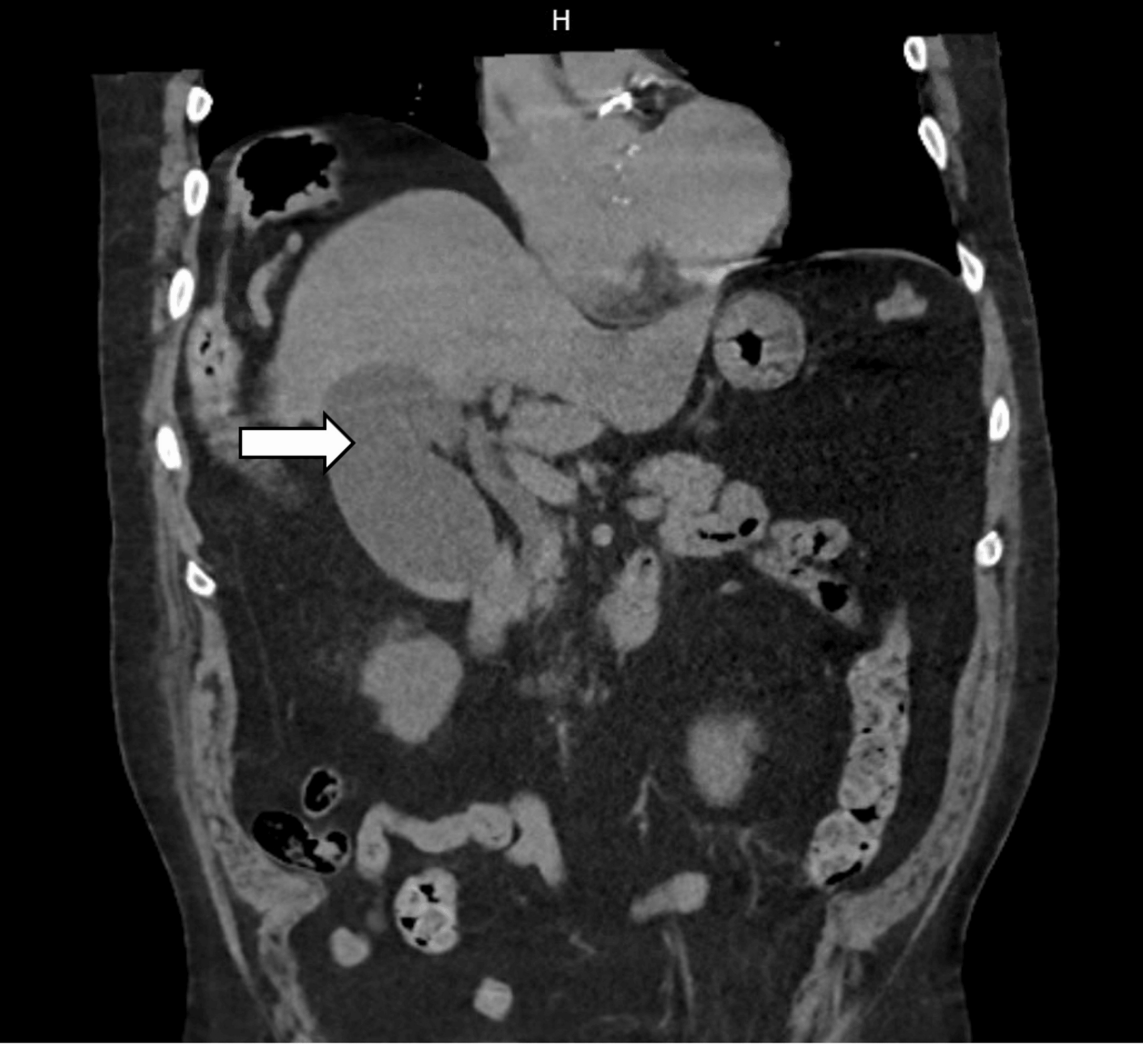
Non-contrast computed tomography (CT) of the abdomen and pelvis showing a distended gallbladder with gallstones.
